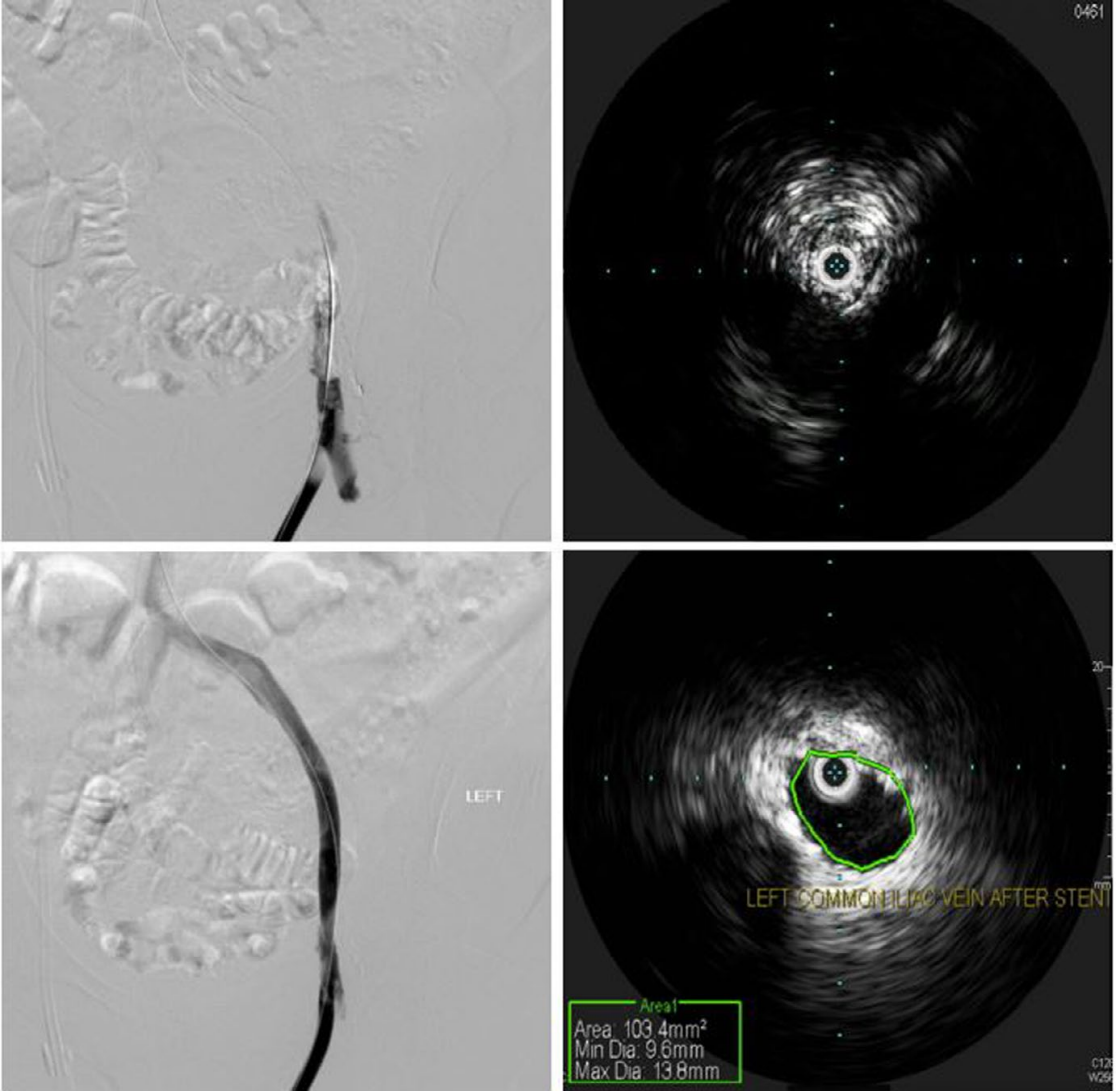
(A) Angiography of lower extremity AV graft showing occlusion of the graft; (B) IVUS showing thrombus occluding the left common iliac vein; (C) Angiography of lower extremity AV graft after thrombectomy with successful blood flow; (D) IVUS showing successful revascularization of left common iliac vein after thrombectomy and stent. AV, arteriovenous; IVUS, intravascular ultrasound
